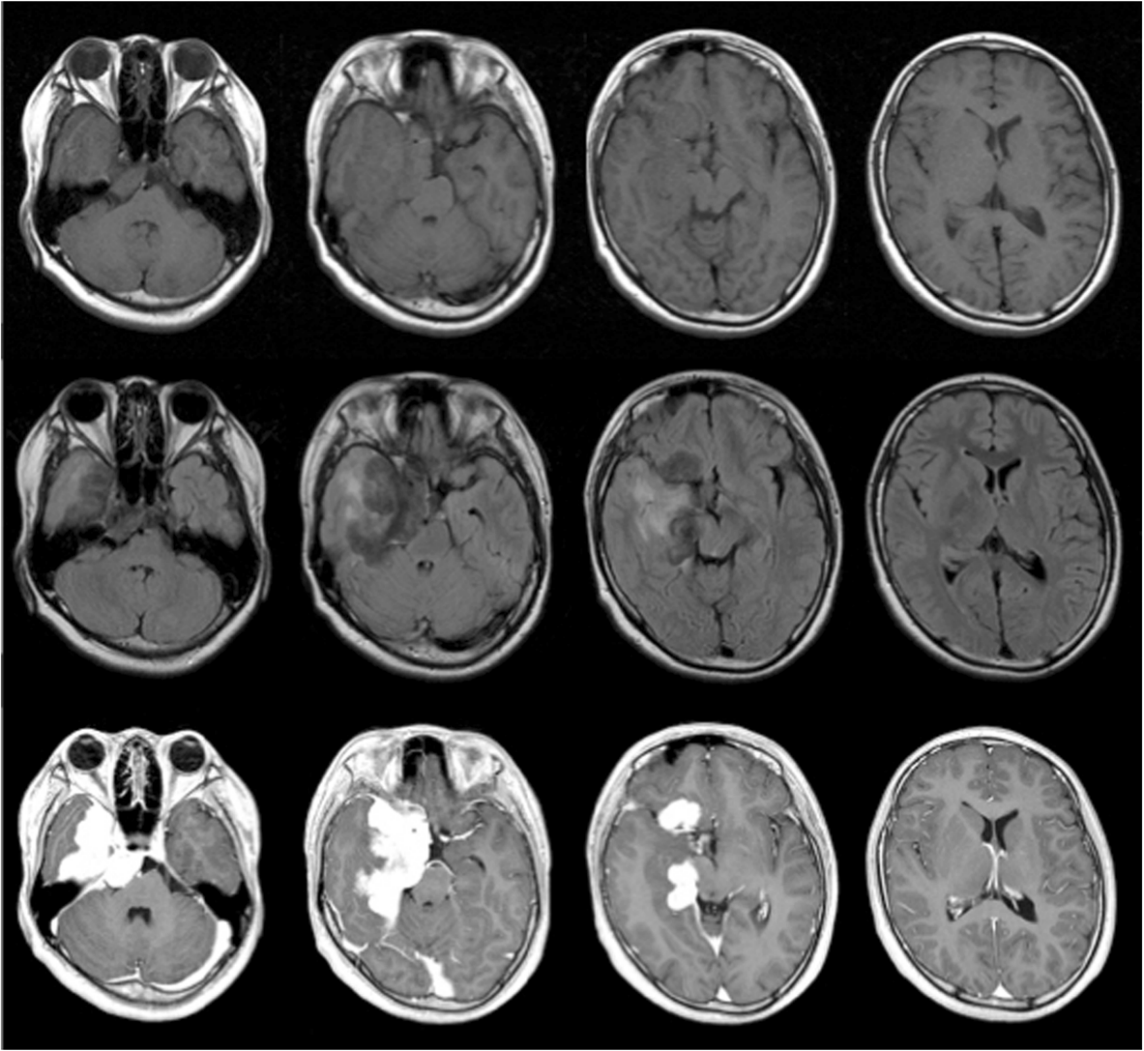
Preoperative axial MRI of the brain. T1-weighted (upper row) and fluid-attenuated inversion recovery (FLAIR; middle row) images demonstrated iso-intensity and a low-intensity mass lesion with edema in the right skull base, respectively. T1-weighted images after administration of gadolinium (lower row) revealed an homogeneous enhanced leaf-shaped huge mass lesion with pachymeningeal and leptomeningeal enhancement involving the tentorium
